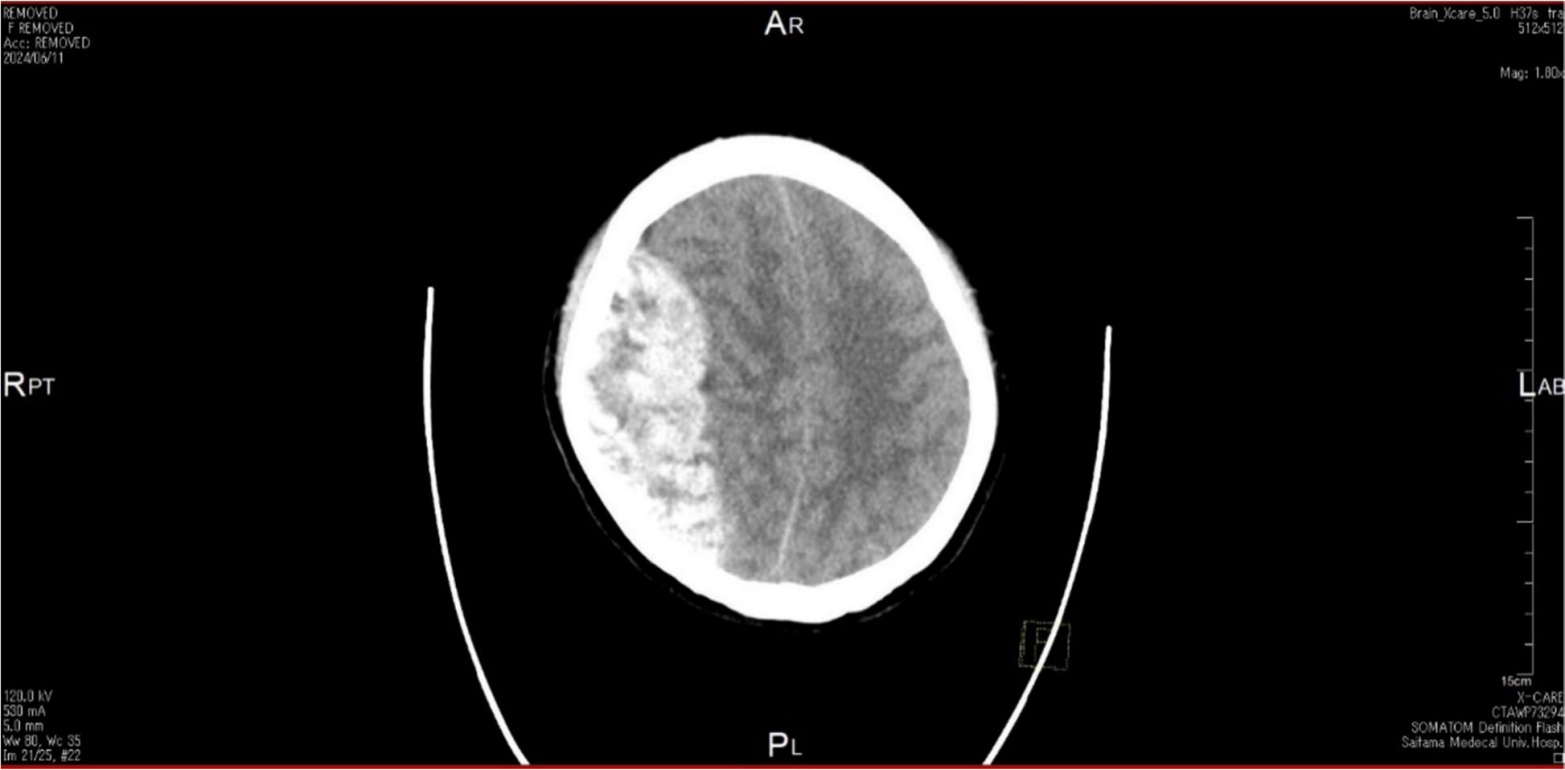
Computed tomography of the head (horizontal) after cesarean section before neurosurgery revealing an epidural hematoma with a midline shift in the right parietal region. Fracture lines are not recognized
